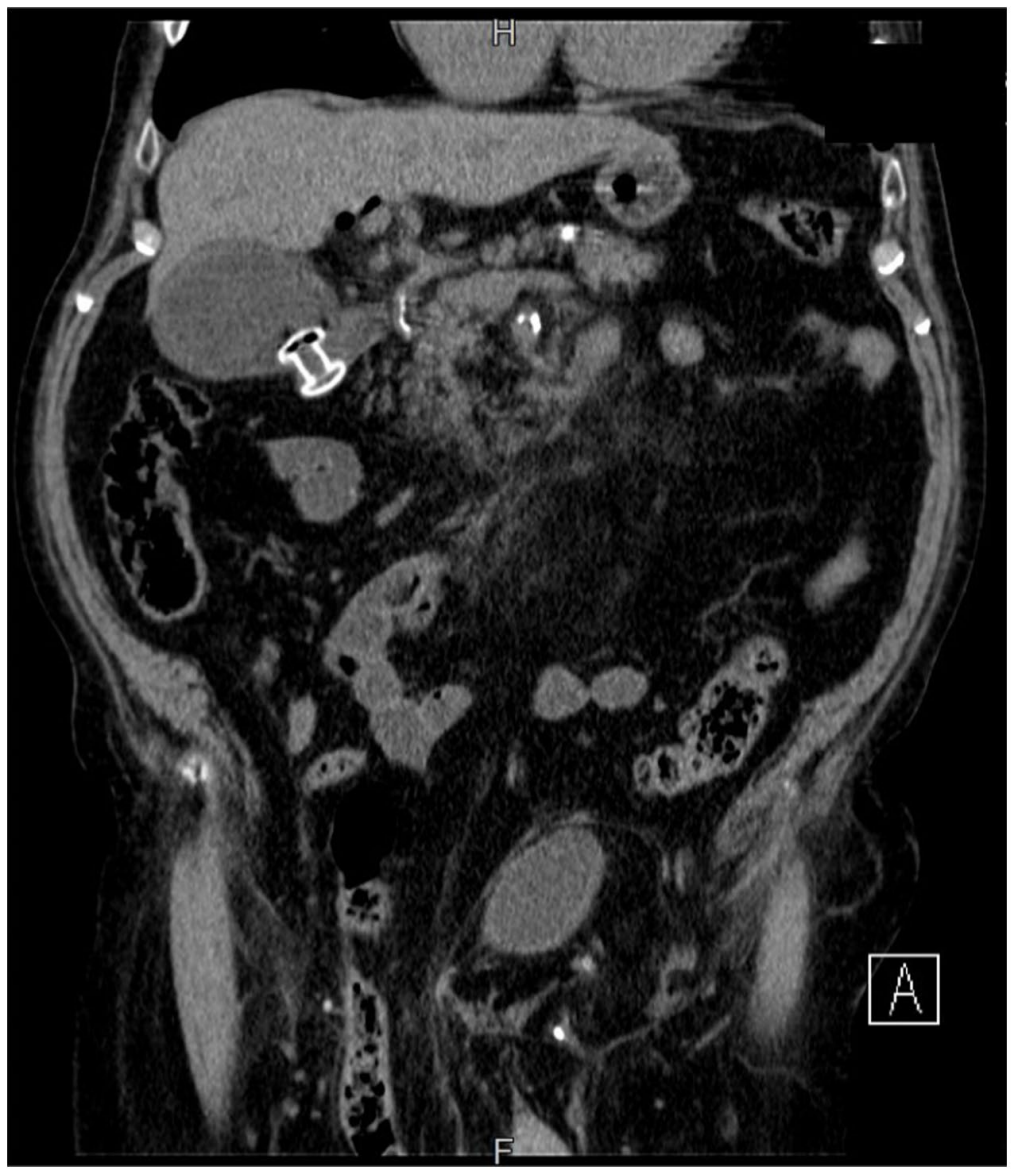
Abdominal computed tomography of EUS-GBD.
